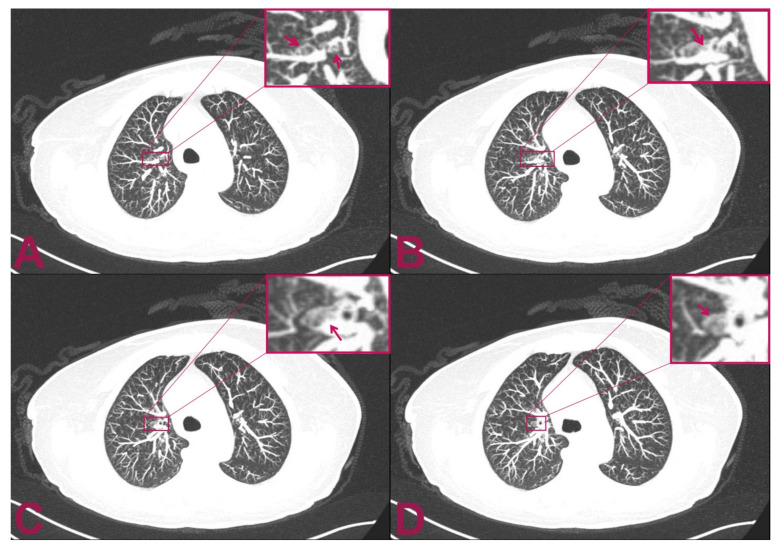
Postoperative multilayer spiral CT scan of the chest with intra-venous administration of contrast medium. The patient is affected by intramedullary cervical metastasis from a primary small-cell neuroendocrine carcinoma of the lung. (A,B) Axial views show two small, rounded, and confluent nodules located at the level of the superior segment of the right upper lobe of the lung which have a maximum diameter of 9 × 3 mm. (C,D) The CT axial view shows an additional 2.5 mm abnormal small, solid nodular formation that is located caudally to the previously indicated nodules. The mass appears adherent to the bronchial tube and measures 8 mm in maximum diameter.
